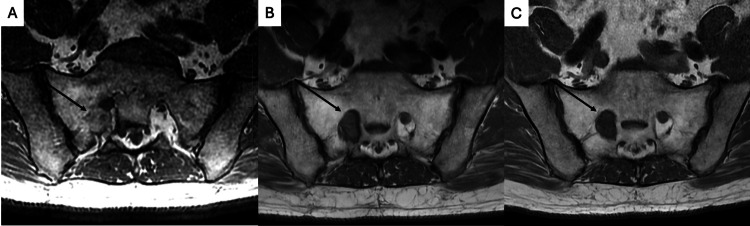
Pre- and post-treatment imaging of the right S1 malignant melanotic nerve sheath tumor (Case 3). Axial pre-contrast T1-weighted MR images, with arrows indicating the tumor, demonstrating the right S1 tumor (A) immediately prior to SRS, (B) 2.6 months after SRS, and (C) 2.4 years after SRS. SRS: stereotactic radiosurgery.
